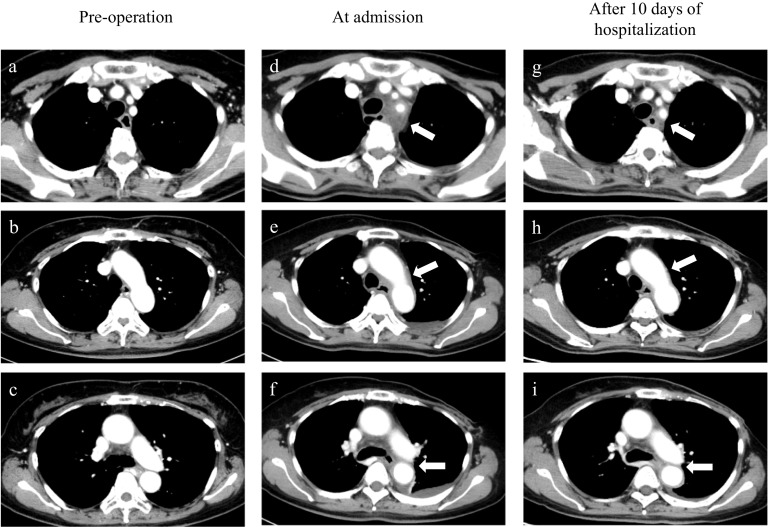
Pre-operation computed tomography (CT) revealed no abnormalities around the aorta (a–c). CT scanning at the time of admission (d–f) revealed thickening of the arterial wall of the thoracic and abdominal aorta and increased fat concentration in the surrounding area. A CT scan after 10 days of hospitalization revealed reduced arterial thickening and fat concentration relative to the CT at the time of admission (g–i)
